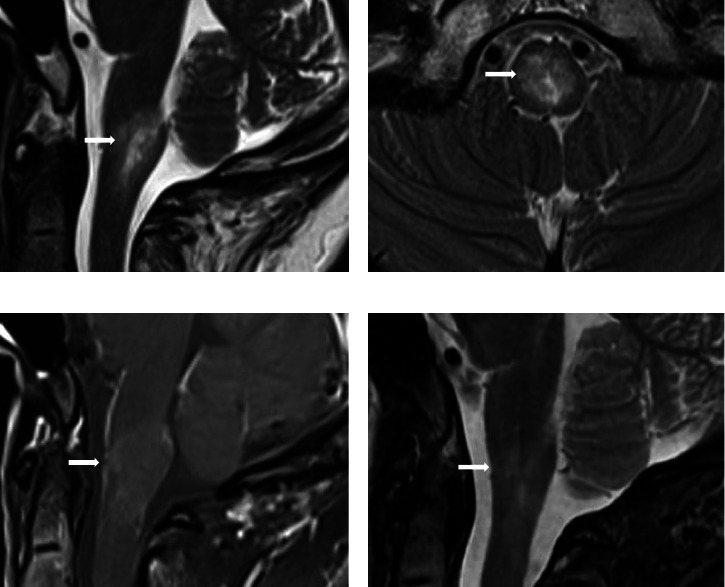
Magnetic resonance imaging (MRI) of the brain. In the first MRI performed to investigate her persistent hiccups and upbeating nystagmus, T2- (a-b) and T1-weighted (c) projections revealed a T2-hyperintense intra-axial lesion in the lower medulla and upper cervical cord (white arrow) which enhances peripherally with gadolinium (c; white arrow). Sagittal T2 projection of another MRI performed 4 months later showed a reduction in the extent of the lesion (d; white arrow).
